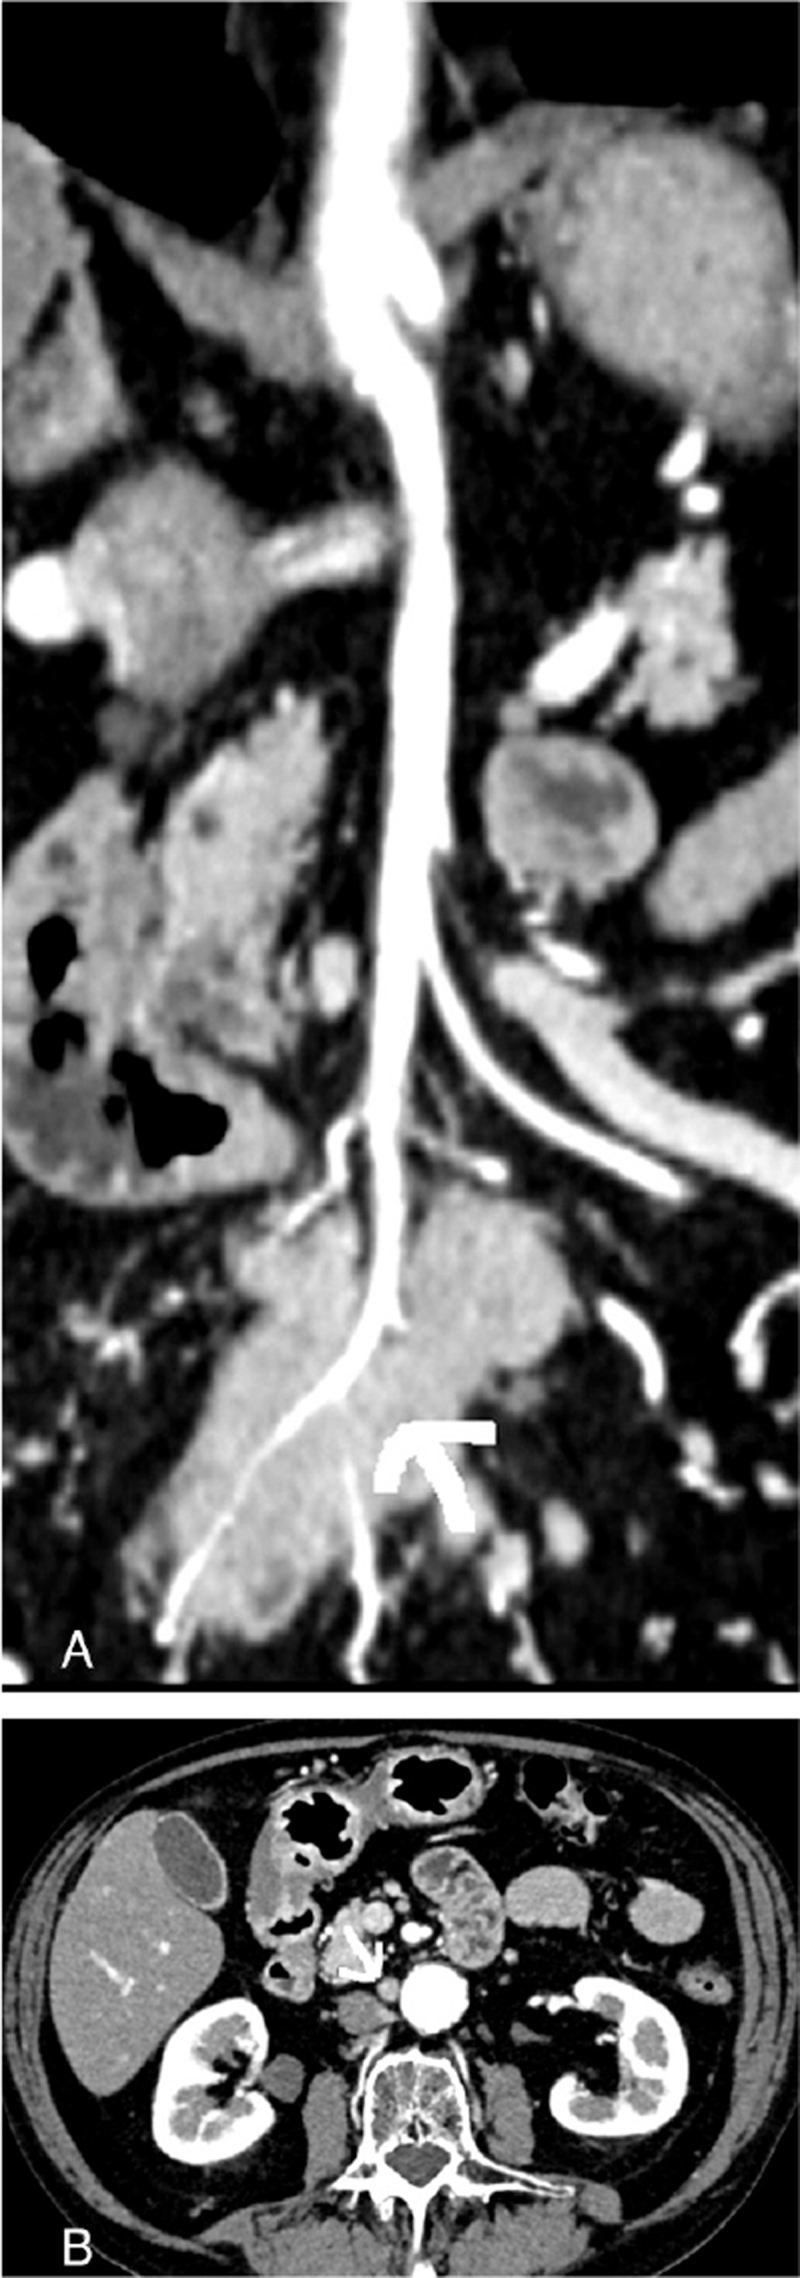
(A) Coronal view CT of tumour (arrow) with vascular reconstruction: encased distal branches of superior mesenteric artery. (B) CT view of interaortocaval lymph node (arrow): enlarged node with short axis of 11 mm. CT = computed tomography.
